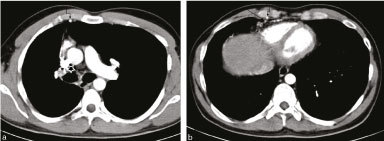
Axial contrast-enhanced CT in the mediastinal window setting revealed prominence of the left PA and its divisions with complete absence of the mediastinal portion of the right PA [
